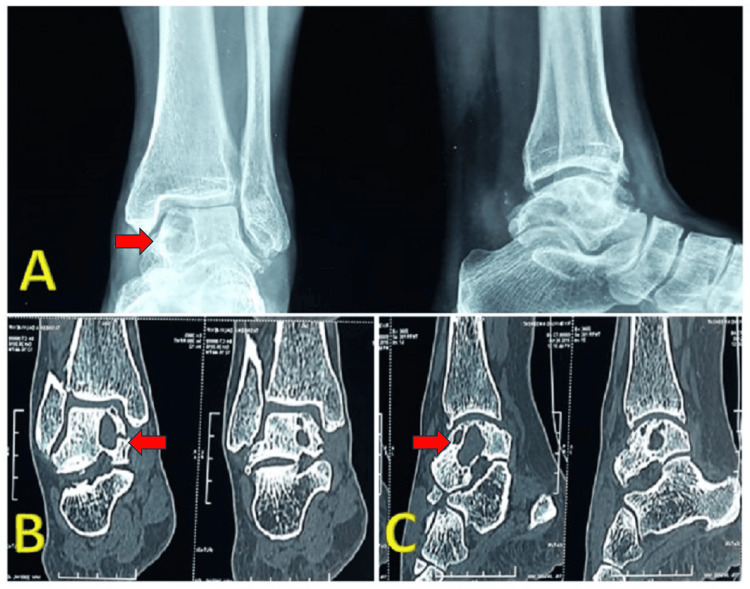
Preoperative Imaging Figure 1A shows preoperative x-ray showing radiolucent regions in the subchondral area of the medial talar dome and body of the talus, with no joint line disruption. Figure 2B (sagittal) and 2C (coronal) CT scan cuts showing radiolucent multiloculated cyst in the medial talar dome and body, without any signs of bone collapse or expansion.
